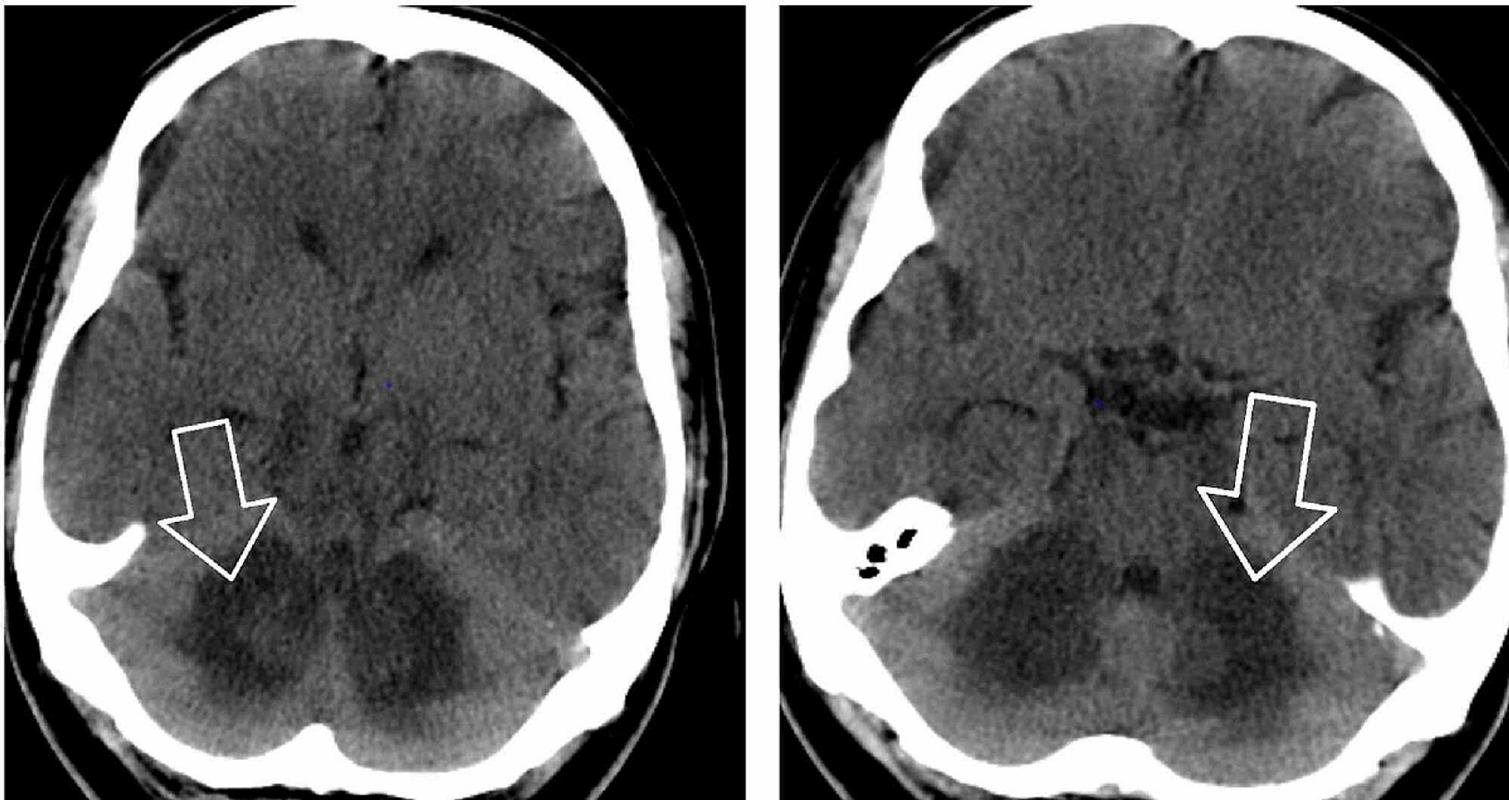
CT head without contrast
